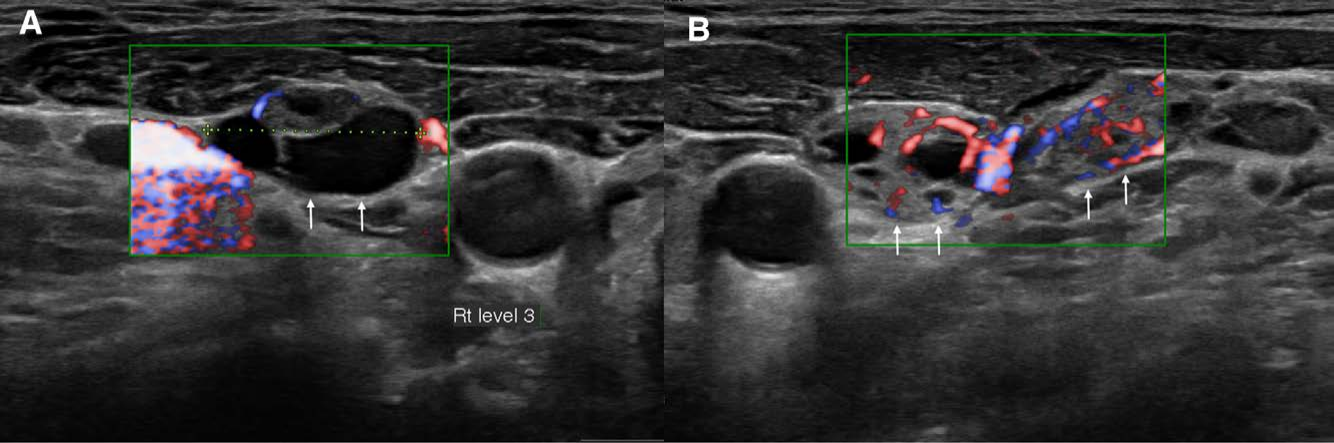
Ultrasonography shows hyperechoic lymph nodes enlargements (arrows) with hypervascularity and cystic changes in the right level III (A) and left level IV (B) regions.
